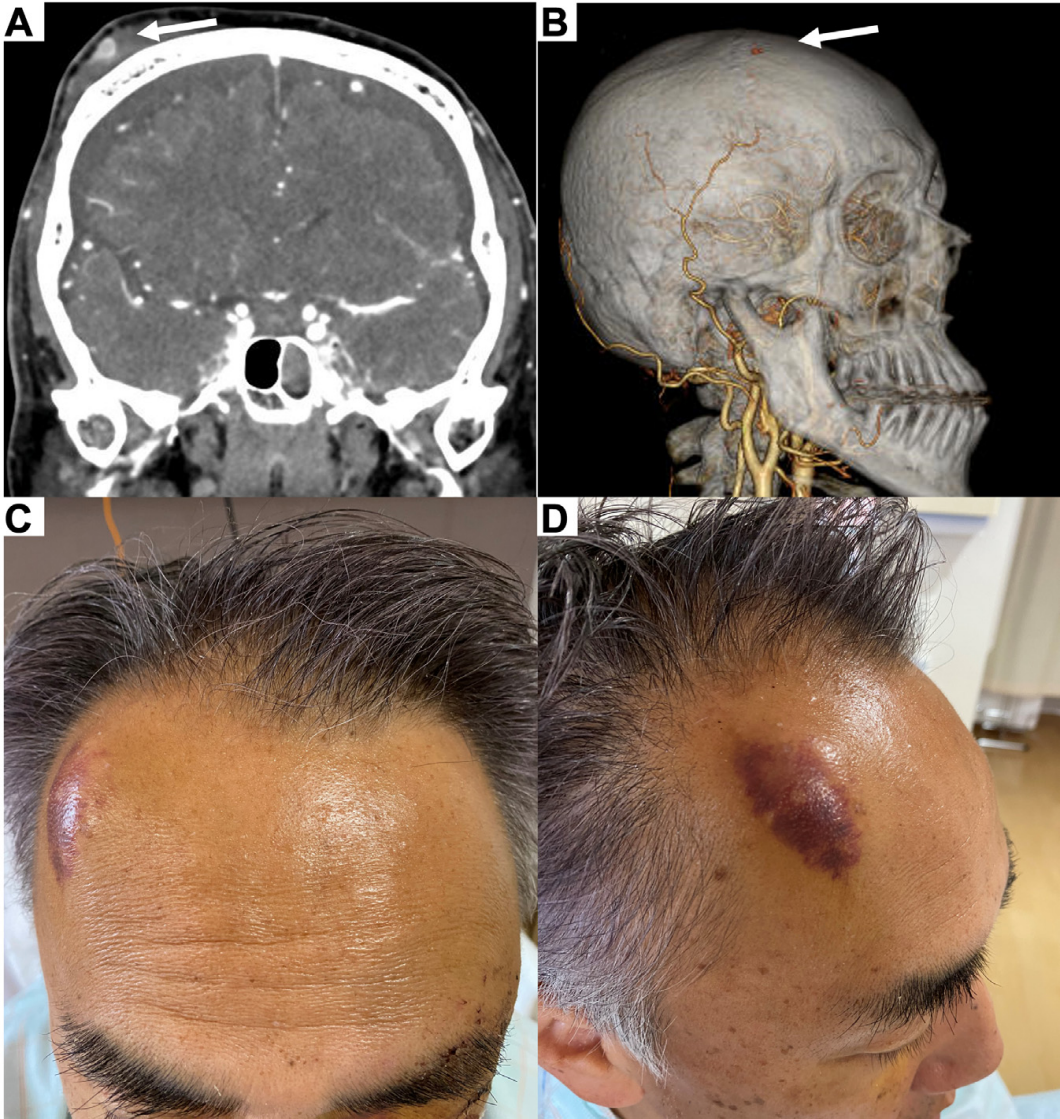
A, Contrast-enhanced computed tomography scan revealing a right frontal subcutaneous hemorrhage with a 9 mm pseudoaneurysm. B, Computed tomography angiography demonstrating a 9 mm pseudoaneurysm at the periphery of the frontal branch of the right superficial temporal artery. C and D, Clinical image of a pulsatile mass that appeared in the right frontal area on the day following admission.
